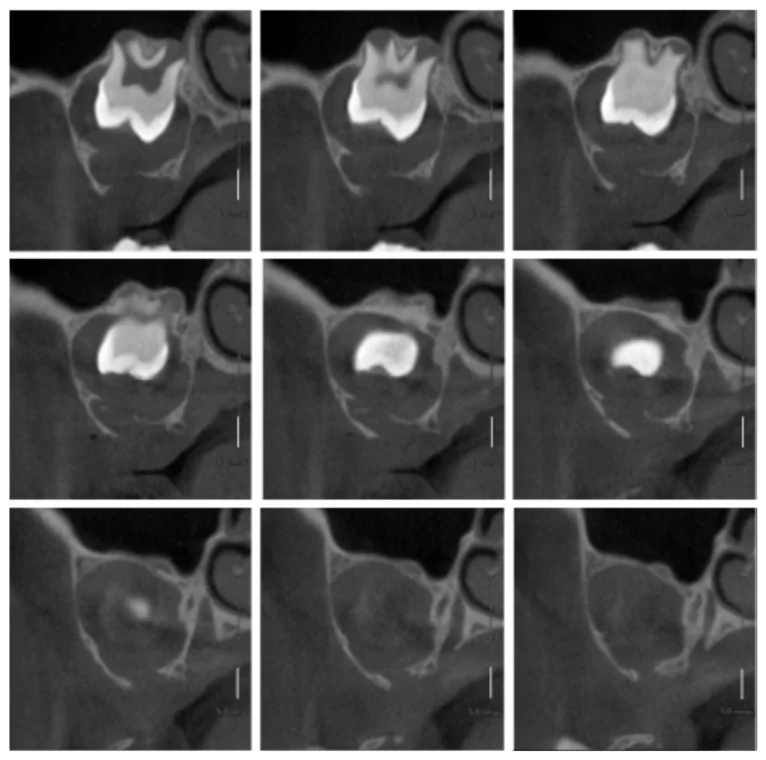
Coronal section of CBCT images at 12 years 6 months of age. Scale bar = 5 mm. Although the second molar was close to the maxillary sinus, a bone of at least approximately 0.5 mm was present. There was no invasion observed into the maxillary sinus.
